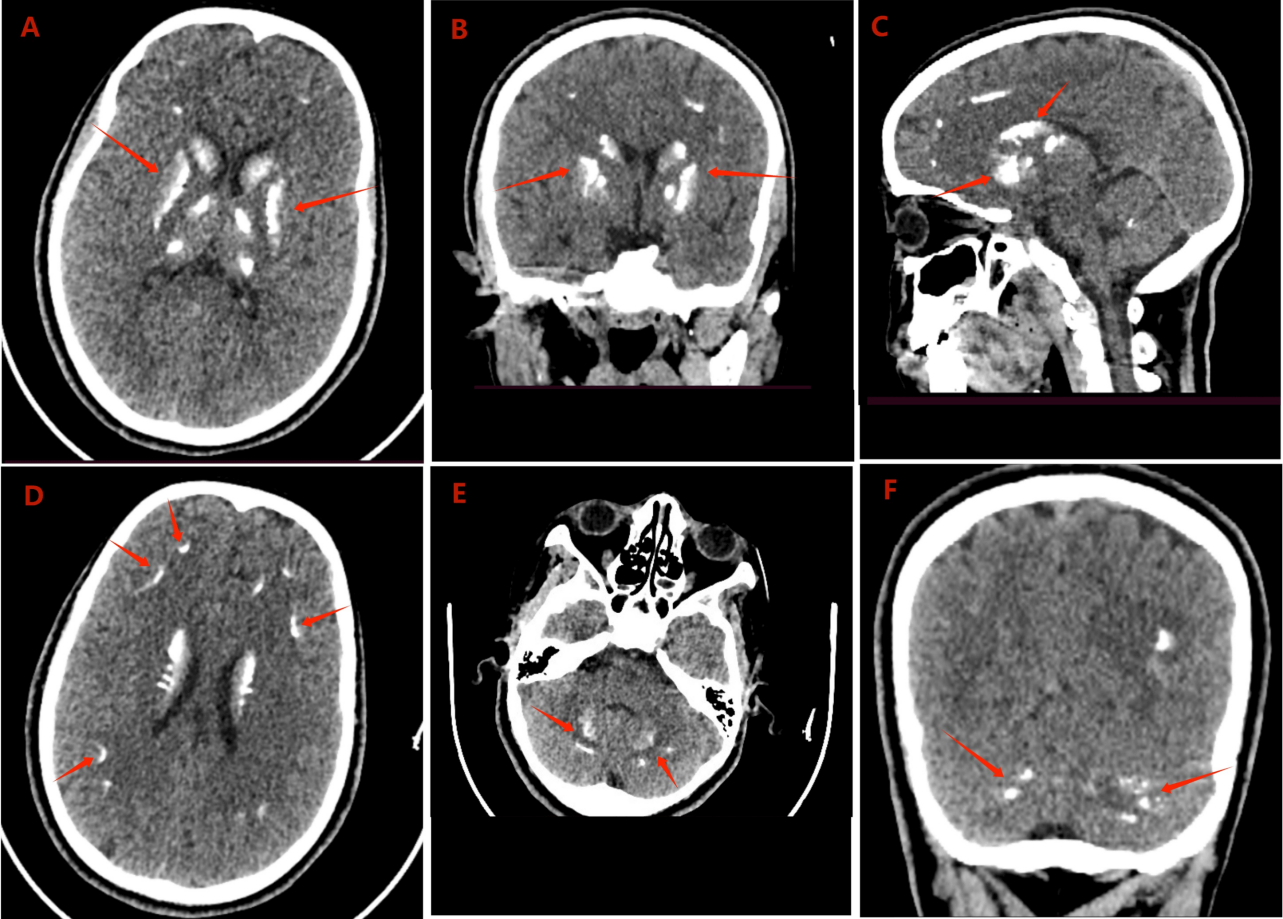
A non-contrast brain CT scan showed bilateral, symmetrical calcifications in the basal ganglia, thalami, corona radiata, and dentate nuclei. (A) Axial, (B) coronal, and (C) sagittal images showing bilateral calcifications of the basal ganglia and thalami (red arrows). (D) Axial image showing bilateral calcifications of the corona radiata (red arrows). (E) Axial and (F) coronal images showing bilateral calcifications of the dentate nuclei (red arrows).
